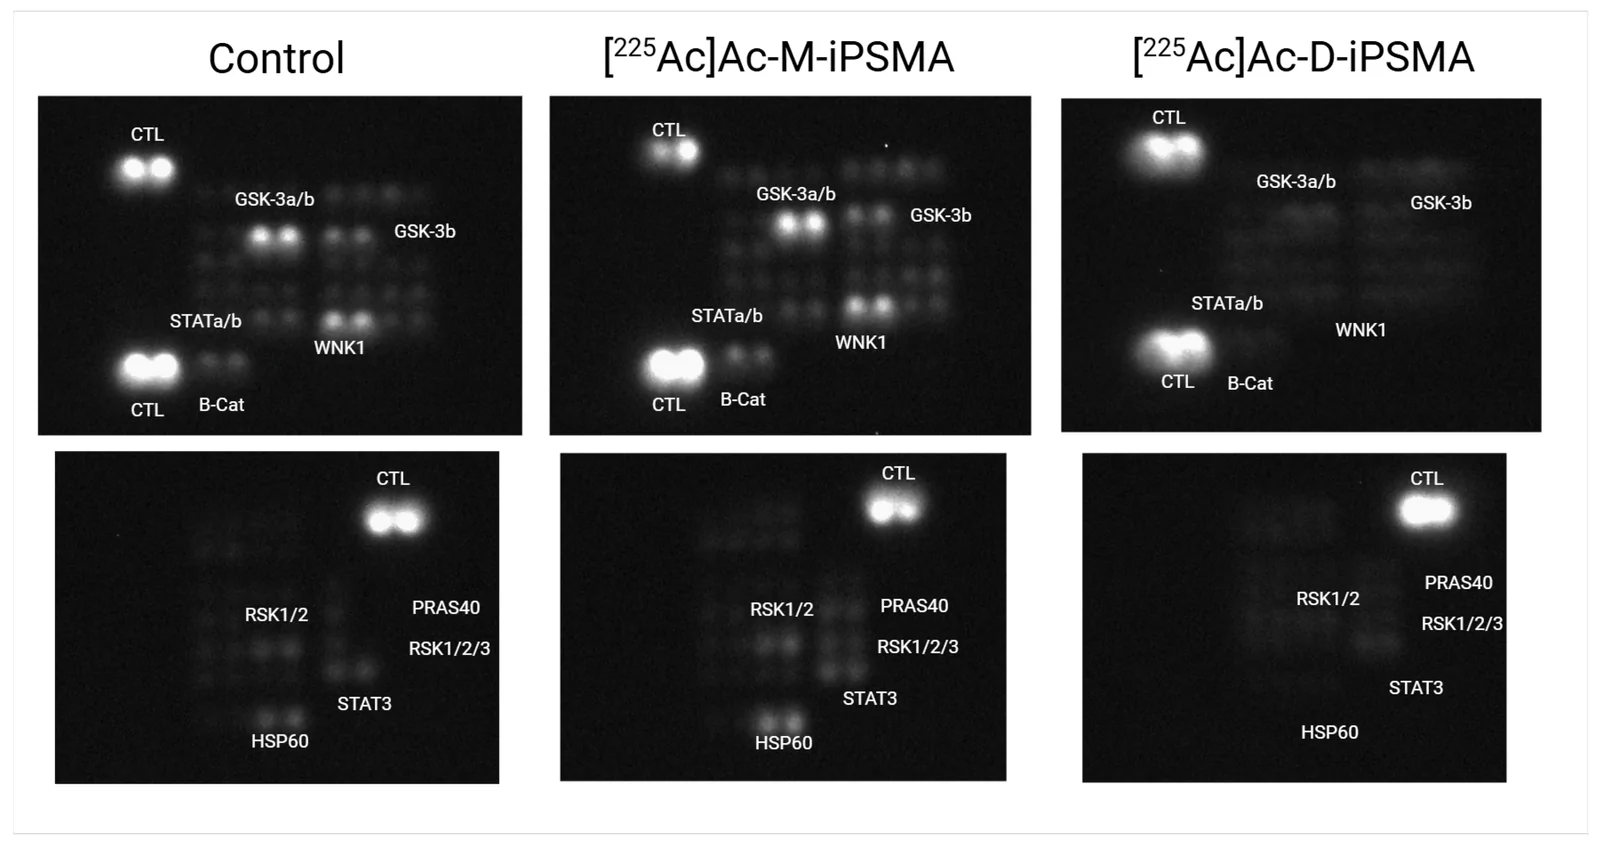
Effect of alpha-based radiopharmaceuticals [^225^Ac]Ac-M-iPSMA and [^225^Ac]Ac-D-iPSMA in human phosphokinase of breast cancer cells. Left panel, phosphokinase array of 4T1 cancer cells without treatment (control). Middle panel, phosphokinase array of 4T1 cancer cells after 4Gy of [^225^Ac]Ac-M-iPSMA. Right panel, phosphokinase array of 4T1 cancer cells after 4Gy of [^225^Ac]Ac-D-iPSMA.
